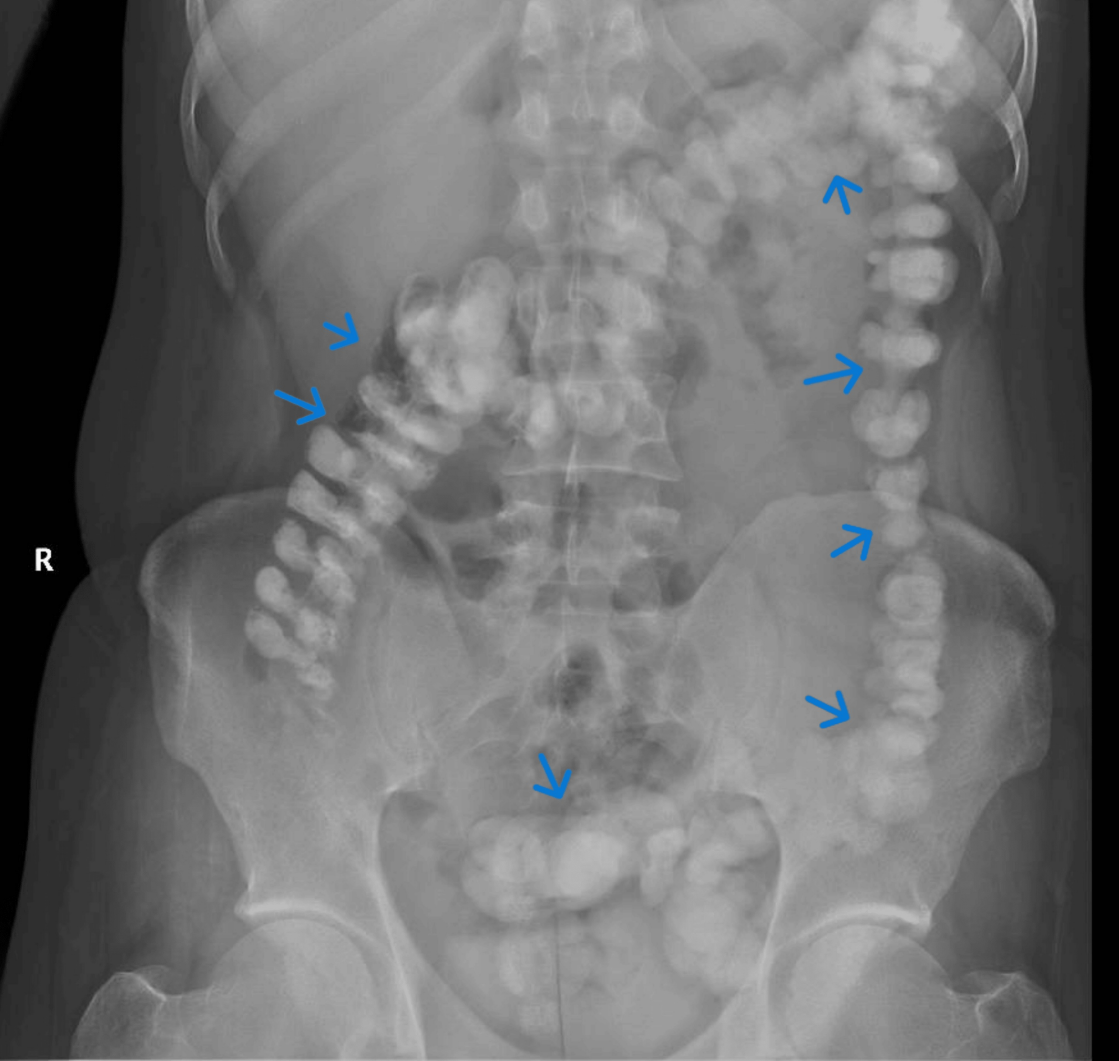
Erect abdominal X-ray done upon admission showing colon filled with stool and stool impaction in the sigmoid (blue arrows indicate stool impaction)
